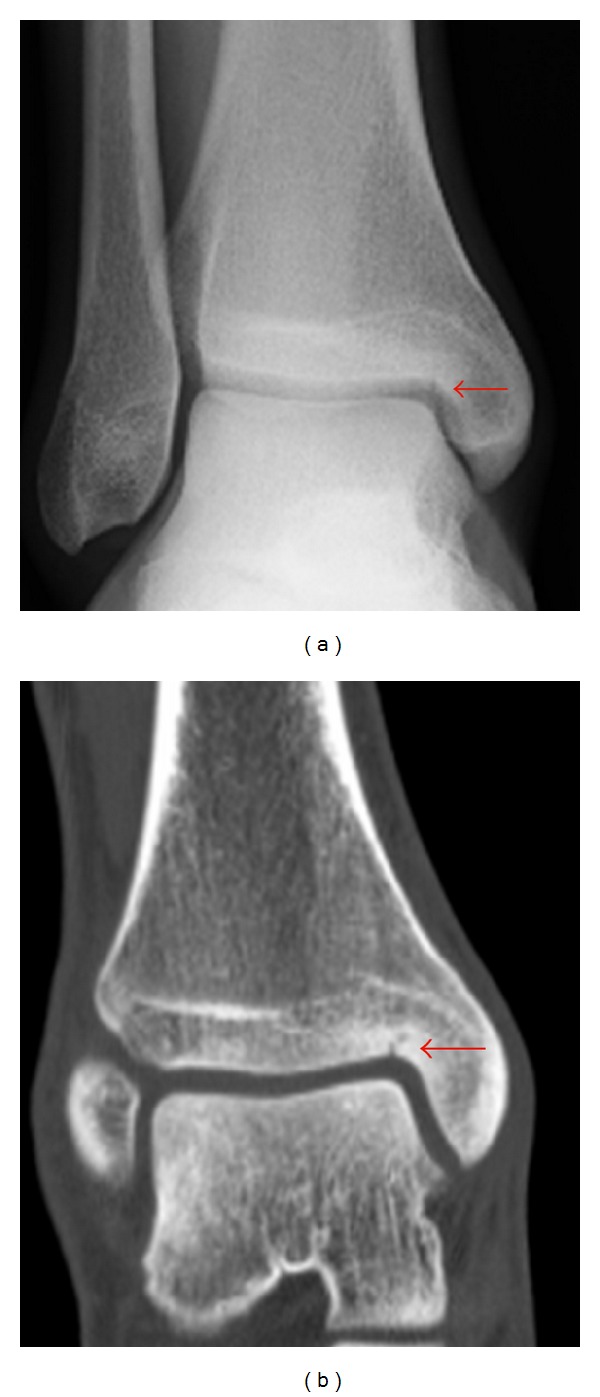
Anteroposterior radiograph (a) and coronal CT image (b) of the right ankle reveal a linear fracture line originating from superomedial corner of the ankle (arrow).
